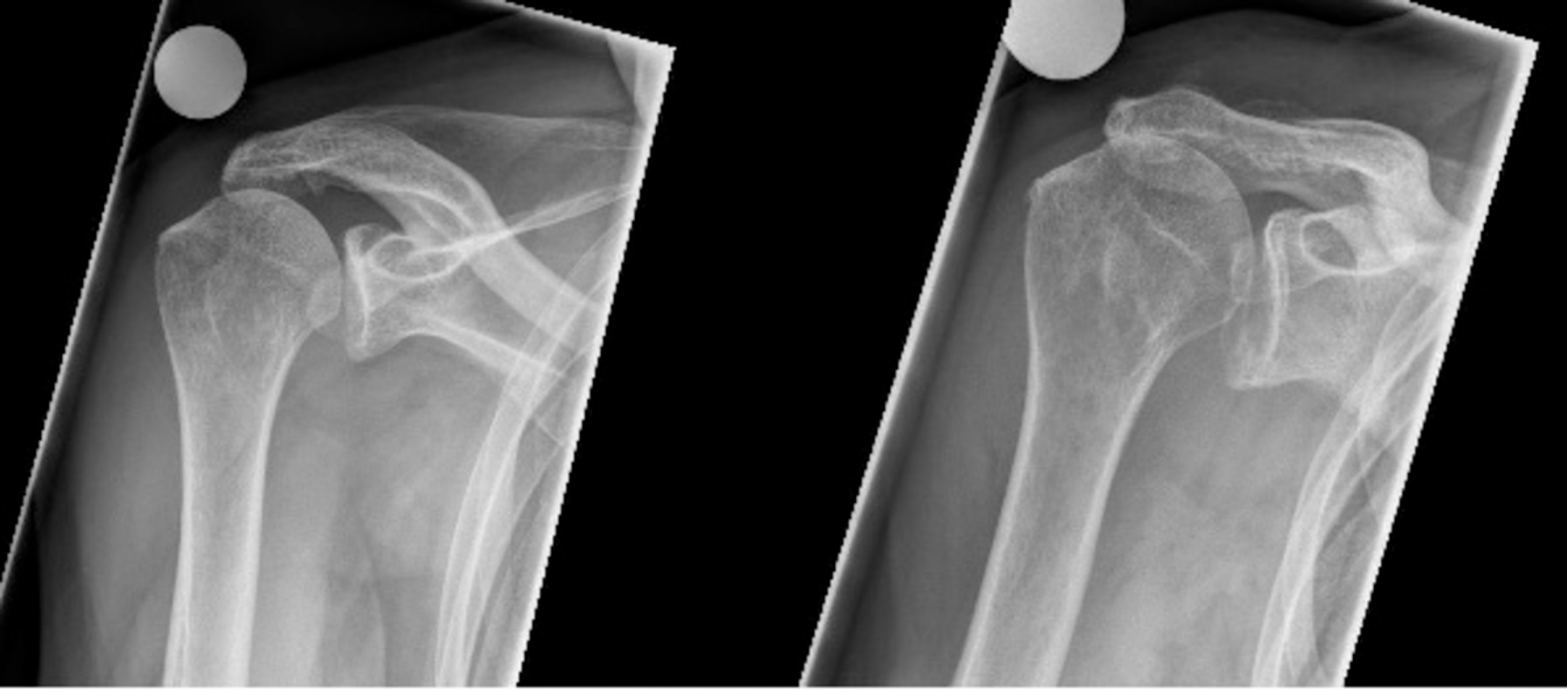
Preoperative (left) radiograph of a 74-year-old male patient with a Hamada grade 2; postoperative (right) radiograph showing a progression of the Hamada grade to 3 after 11 years.
